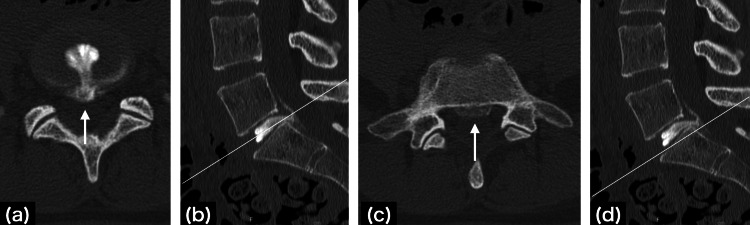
Post-discoblock computed tomography (a) Axial view at the level of (b), (b) midline sagittal view, (c) axial view at the level of (d), and (d) midline sagittal view. A lumbar disc herniation protruding centrally (white arrows) is observed showing migration both upward and downward and is a contained type that does not penetrate the posterior longitudinal ligament.
